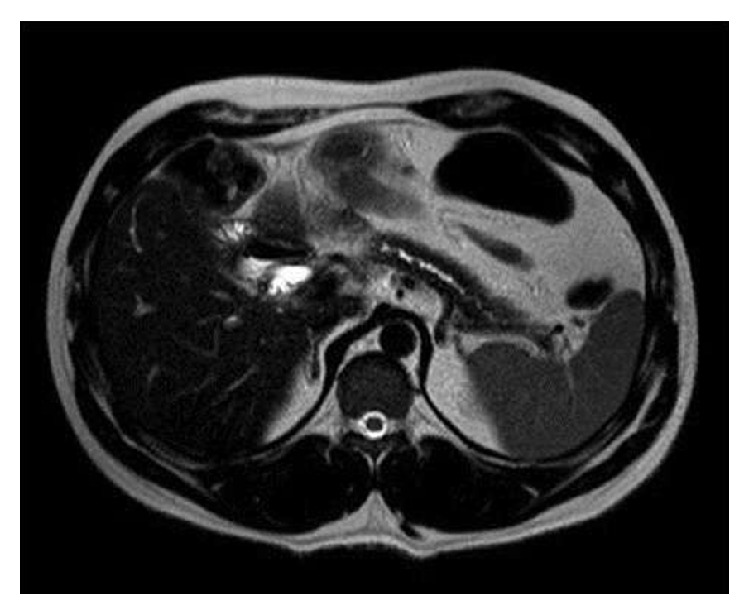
MRI shows main pancreatic duct dilation and stones.
